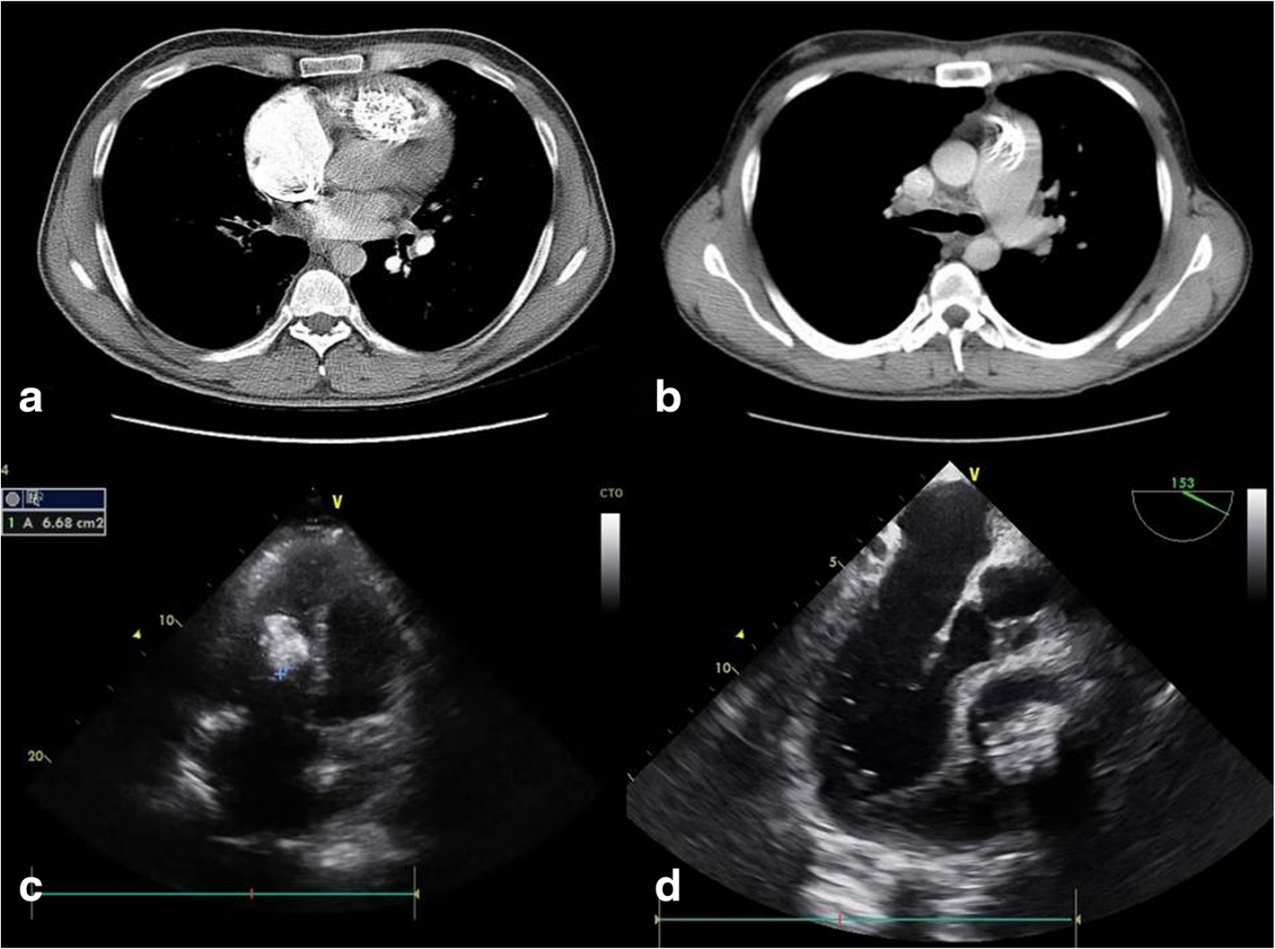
a Chest computed tomographic scan demonstrates an approximately 4 × 10–cm calcified mass in the right ventricle attached to the pulmonary valve. b Thrombosis of the origin of the right pulmonary artery is visualized with uniform contrast enhancement of the pulmonary trunk and the left pulmonary artery. c Transthoracic echocardiographic study and d transesophageal echocardiographic scan reveal a calcified mass in the right ventricle attached to the pulmonary valve
